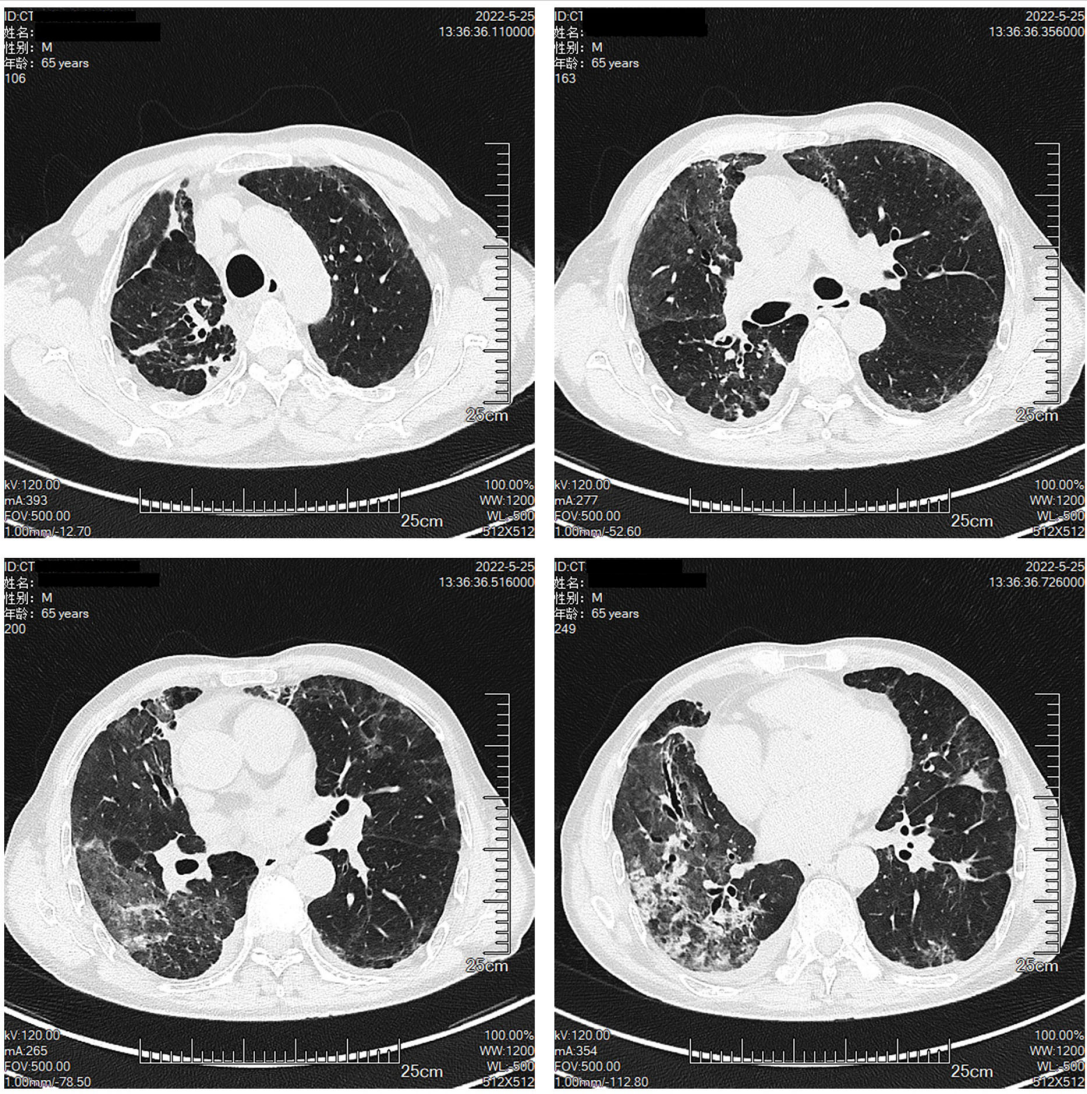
The Chest CT on May 25th.
